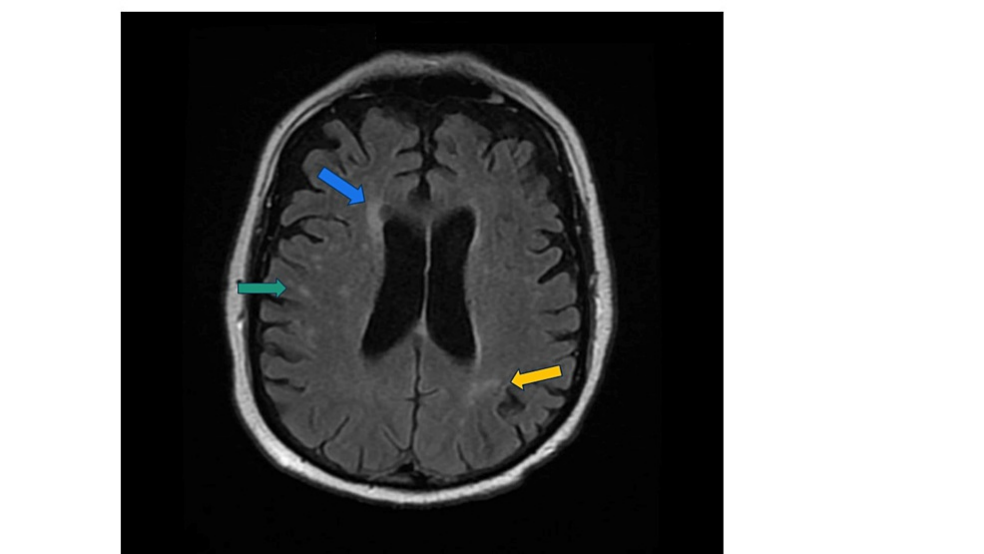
MRI brain without contrast showing bilateral (notably right>left), periventricular (blue arrow), parietal (green arrow), temporal (not shown here), and occipital (yellow arrow) lobe hypo-densities, representative of thromboembolic cerebrovascular accident.
